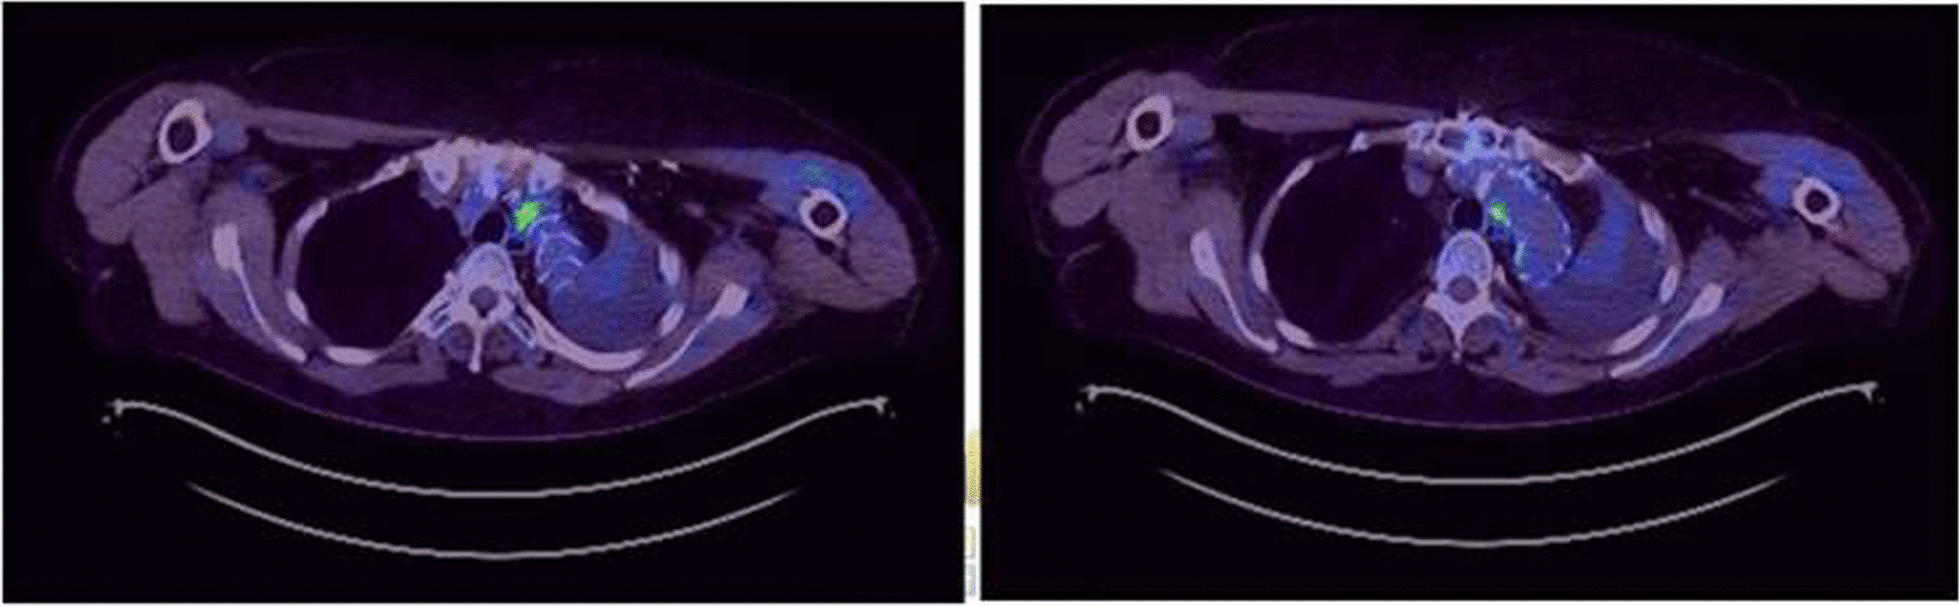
Axial slices of PET CT in March 2022 showed foci of increased uptake concerning for a graft infection. Ongoing complete left sided collapse of the lung is also present
